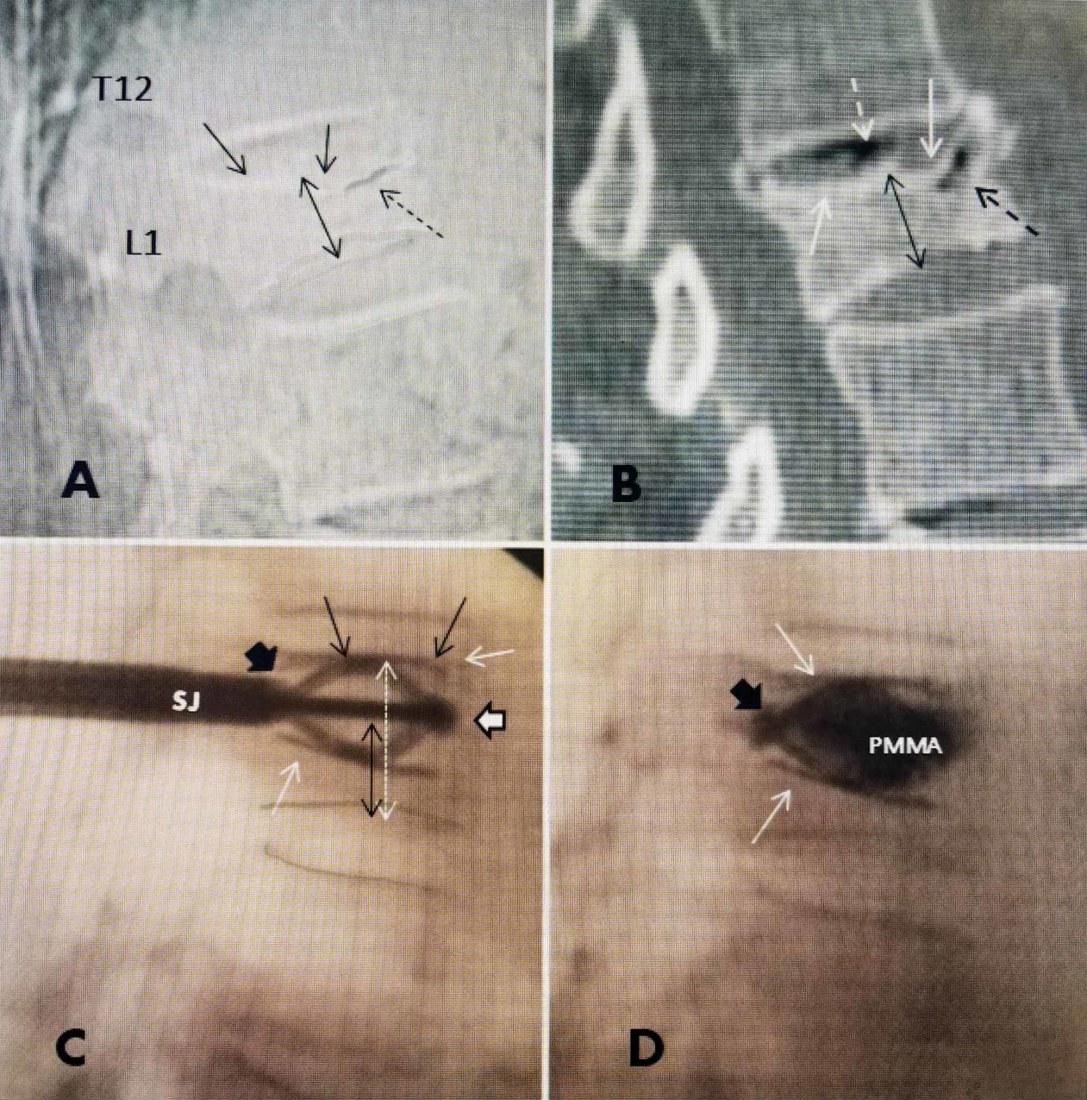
Comparison of preoperative lateral radiograph, sagittal CT scan, and intra-operative films after SpineJack deployment, both before and after cement injection, demonstrating restoration of height of L1 fracture by the implant. A: Lateral plain radiograph showing superior endplate collapse at L1 (solid black arrows) and anterior superior vertebral cleft in the vertebral body just under the fractured endplate (dashed black arrow). The mid-vertebral collapse is marked showing 50% collapse of L1 (double-headed black arrow). B: Sagittal CT scan with bone window demonstrating vacuum cleft in T12-L1 disc space (dashed white arrow) with an additional small vacuum cleft, that can be a sign of osteonecrosis, anteriorly and superiorly in T12 (dashed black arrow). The mid-vertebral height is marked (double-headed fine dashed arrow) indicating almost 50% compression from the collapse of both superior and inferior endplates. C: Lateral intra-operative fluoroscopic film showing the SJ inserted and deployed before the cement is injected. The implant insertion tip is seen anteriorly (solid large white arrow with black border). The struts or wings are deployed and pushing up under the superior endplate (solid black arrows). The inferior strut is restoring support inferiorly (solid white arrow). The original compressed height of the mid-vertebra is indicated (double headed dashed black arrow) compared to the expanded height (double-headed white arrow). D: Lateral film after cement (PMMA) injected through SJ, and the cement is seen to be mainly confined within the boundaries of the implant, with minor spread posteriorly along the connection, just ventral to the base of the pedicle, which is between the insertion handle and the implant (solid large black arrow). The fully extended inferior and superior struts can be seen (solid white arrows) in the same position as before cement injection in 'C'. The four struts are able to broadly re-distribute the load along both superior and inferior endplates and combined with midline PMMA, effectively across the endplates.
